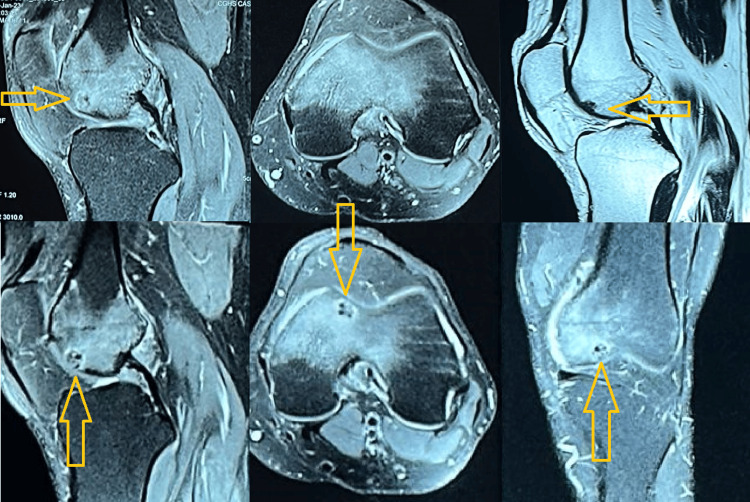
MRI showing the left knee joint Arrows showing the lesion
